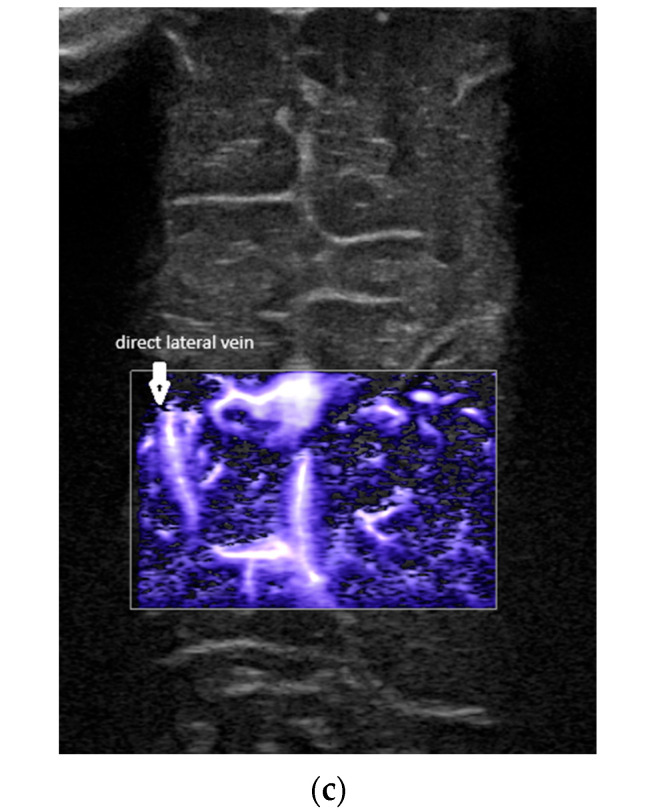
(a) Abnormal venous pattern (colour Doppler). The confluence between the terminal and the internal cerebral vein is identified only on the left (yellow arrow). (b) Abnormal venous pattern (microvascular imaging (MVI)); same view. Confluence between TV and ICV identified on the left only (yellow arrow). (c) Abnormal venous pattern (microvascular imaging (MVI)). A right direct lateral vein with an increased diameter can be observed on the side where the terminal vein is absent.
